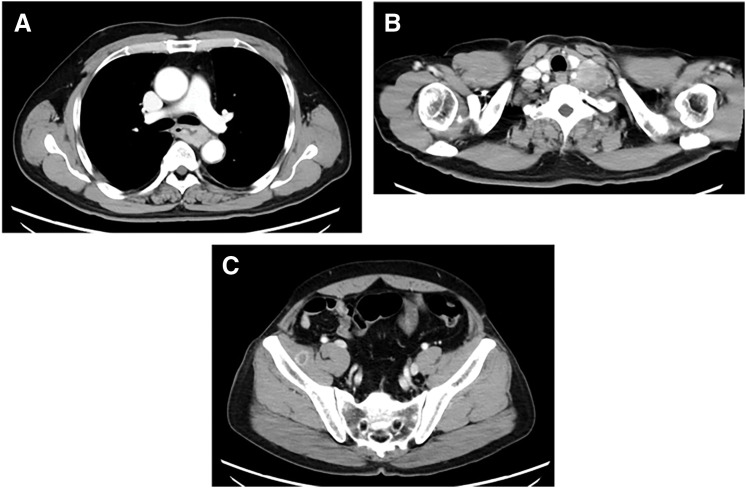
Staging contrast-enhanced CT at initial diagnosis. (A) Axial CT demonstrates an enhancing mass with marked circumferential wall thickening in the middle thoracic esophagus. (B) Axial CT shows left supraclavicular lymph node metastasis (station 104 L). (C) Axial CT demonstrates a metastatic lesion within the iliopsoas muscle.
